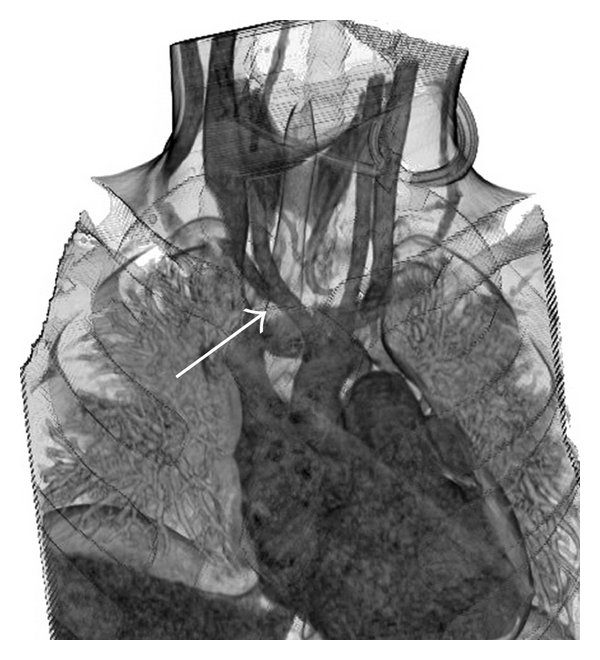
3D CT. Three-dimensional CT shows the innominate artery (white arrow) crossing the trachea from left to right just cranial to the manubrium.
